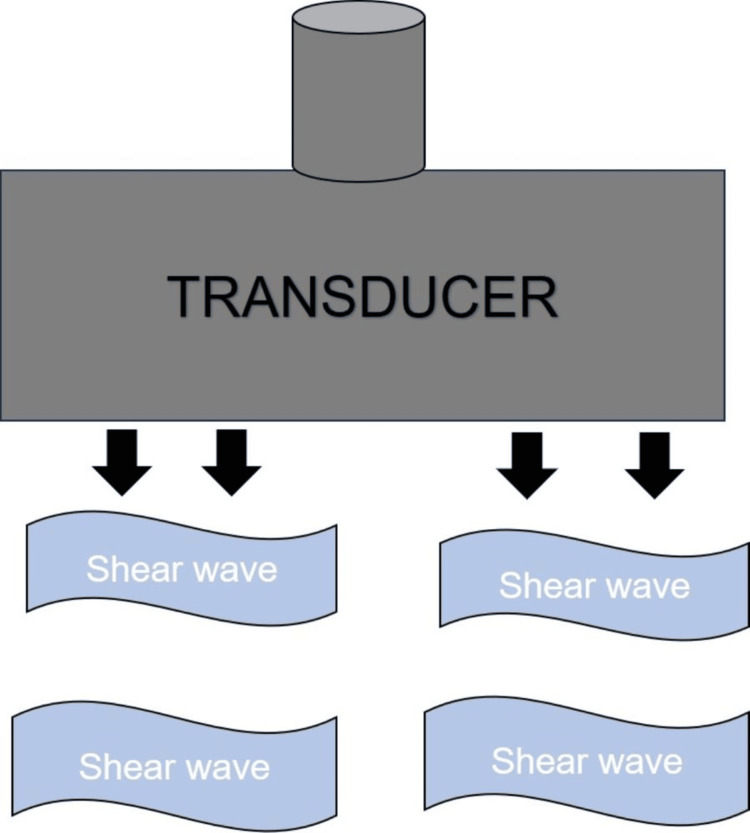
Pictorial description of generation of shear wave by the ultrasound transducer Image credits: Dr. Deepa J. Patil
